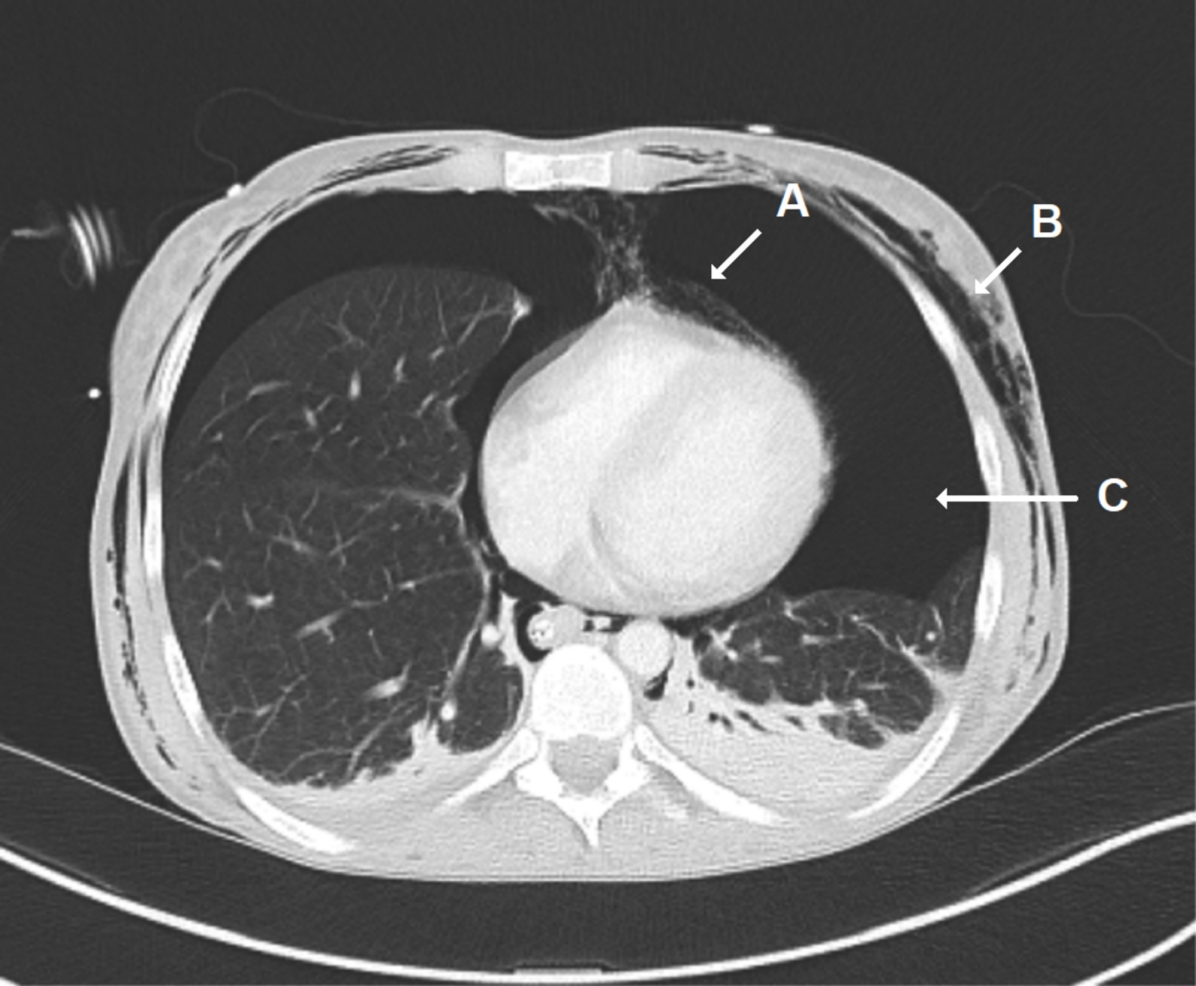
CT of the Chest CT of the chest with contrast, in the axial plane, revealing pneumomediastinum (A), diffuse subcutaneous chest wall emphysema (B), and pneumothorax (C).
